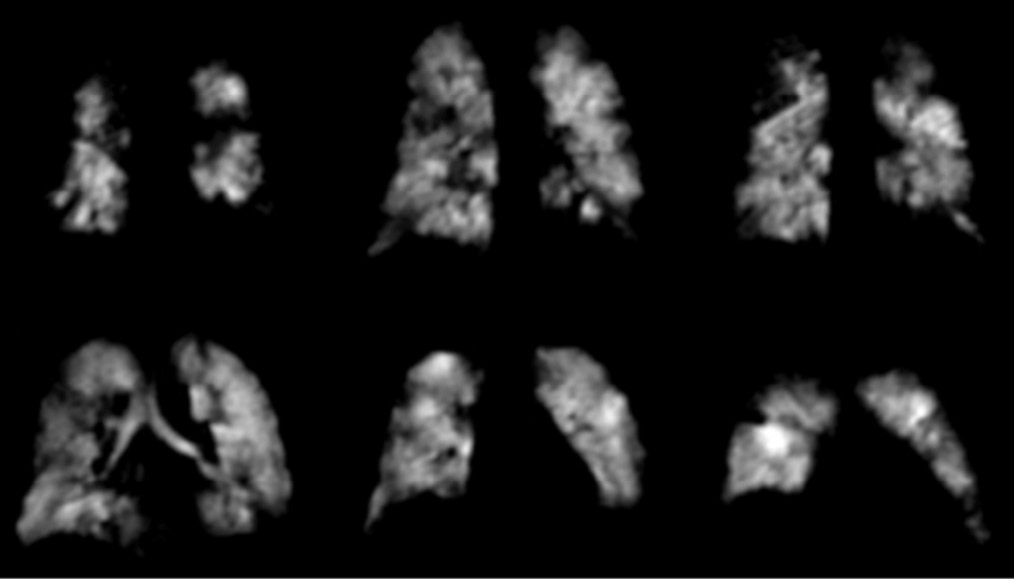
Patient G: difficult asthma in a patient with normal spirometry. Patient was diagnosed with atopic eosinophilic asthma with a high symptom burden yet normal spirometry. Medications at the time of the clinically requested MRI scan were extensive; including mepolizumab 100 mg every 4 weeks, flutiform 250/10 two puffs twice a day, prednisolone 5mgs once daily and tiotropium. Mepolizumab was seen to somewhat alleviate their asthma symptoms and reduced the number of courses of steroids resulting in a marked improvement in quality of life. However, a lower zone wheeze remained and despite long-term steroids, they still required 4—5 rescue courses of additional prednisolone in the previous 2 months. Ventilation MRI showed small and moderate defects and as a result, the decision was made to intensify patient therapy.
